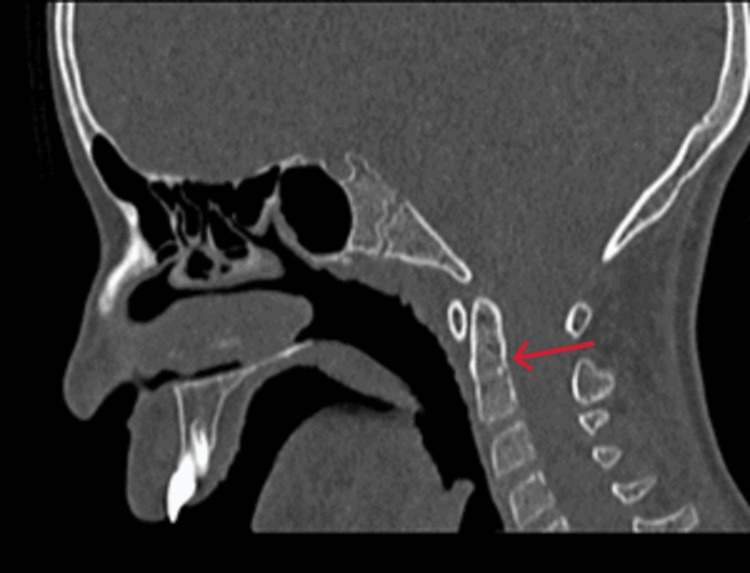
CT of the paranasal sinus sagittal view showing the fusion of C2–C3 cervical vertebrae (marked by a red arrow)
